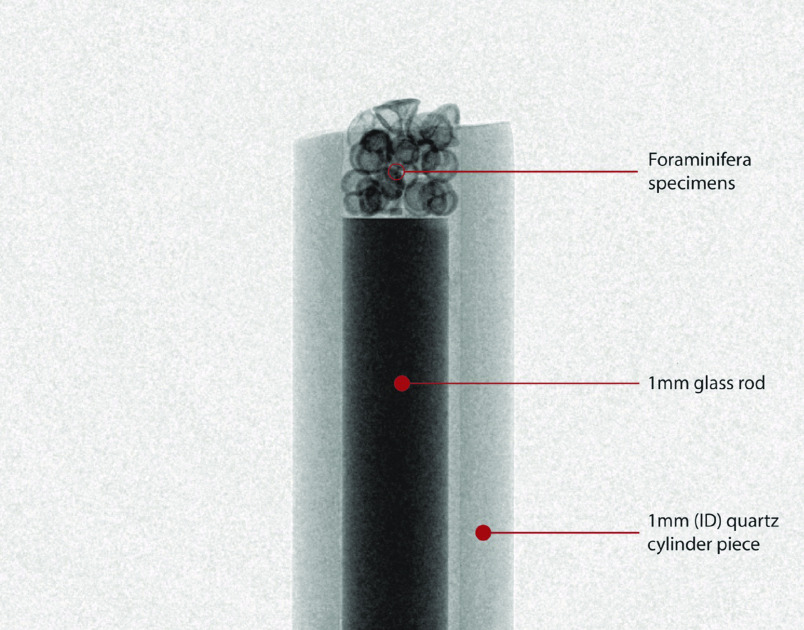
Customized sample container for foraminifera X-ray computer tomography analysis.
